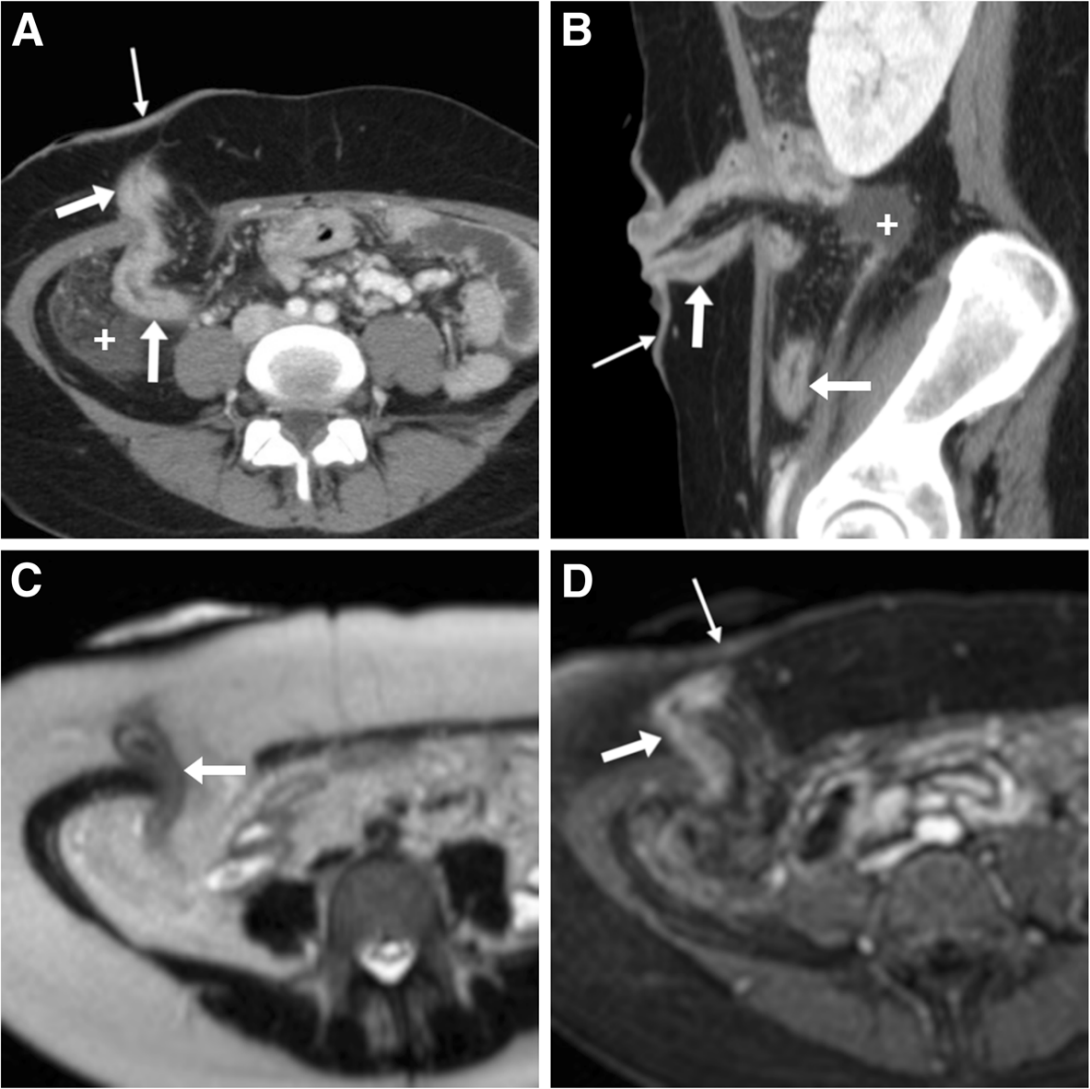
Reactivation of Crohn’s disease at an ileostomy. a, b CT showing diffuse, mildly irregular mural thickening with marked contrast enhancement along the stomal tract and the distal small bowel, consistent with active disease (also note fat stranding and fluid in the right parietocolic gutter). c, d In the same patient, MR-enterography confirms thickened walls on T2-weighted (c) with hyperenhancement on post-gadolinium fat-suppressed (d) images. Additionally, note enhancing inflamed peristomal skin (thin arrows in a, b and d)
